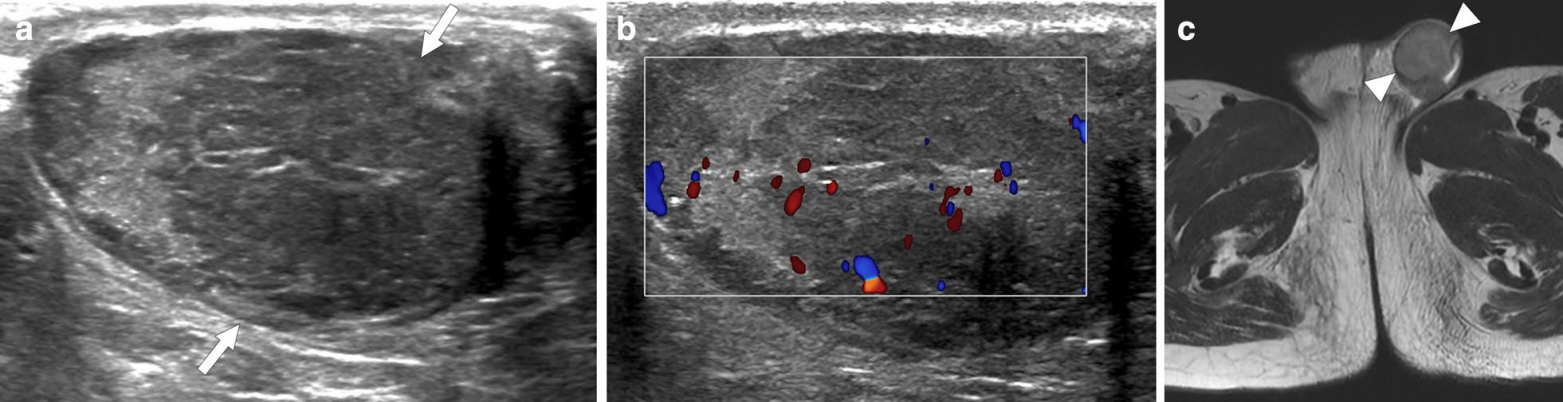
Tuberculous orchitis. A 13-year-old male with known inherited interleukin-12 receptor deficiency and recently diagnosed disseminated tuberculosis. Patient also complained of mild left testicular pain and asymmetric enlargement at his initial presentation. a Gray scale US image shows ill-defined heterogeneous hypoechoic areas (arrows) almost completely replacing the parenchyma. b Color flow Doppler US demonstrated mild-to-moderate vascularity within these hypoechoic areas. c Axial T2-weighted MR image shows enlarged left testis with irregular borders and low T2 signal intensity (arrowheads). Based on the clinical, microbiological and radiological findings, the putative diagnosis was tuberculous orchitis. Serial follow-up US examinations after the start of antituberculosis treatment demonstrated gradual testicular atrophy
